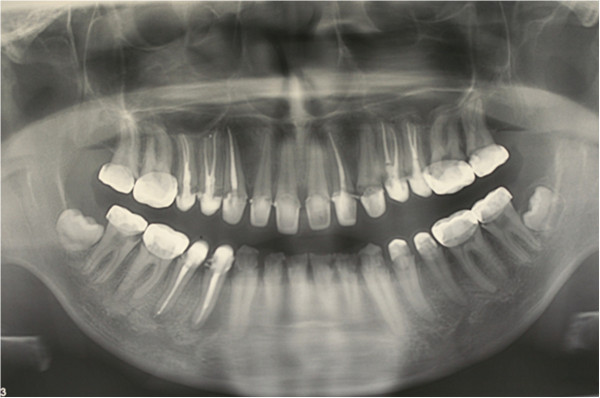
Post-endodontic treatment panoramic radiograph.
